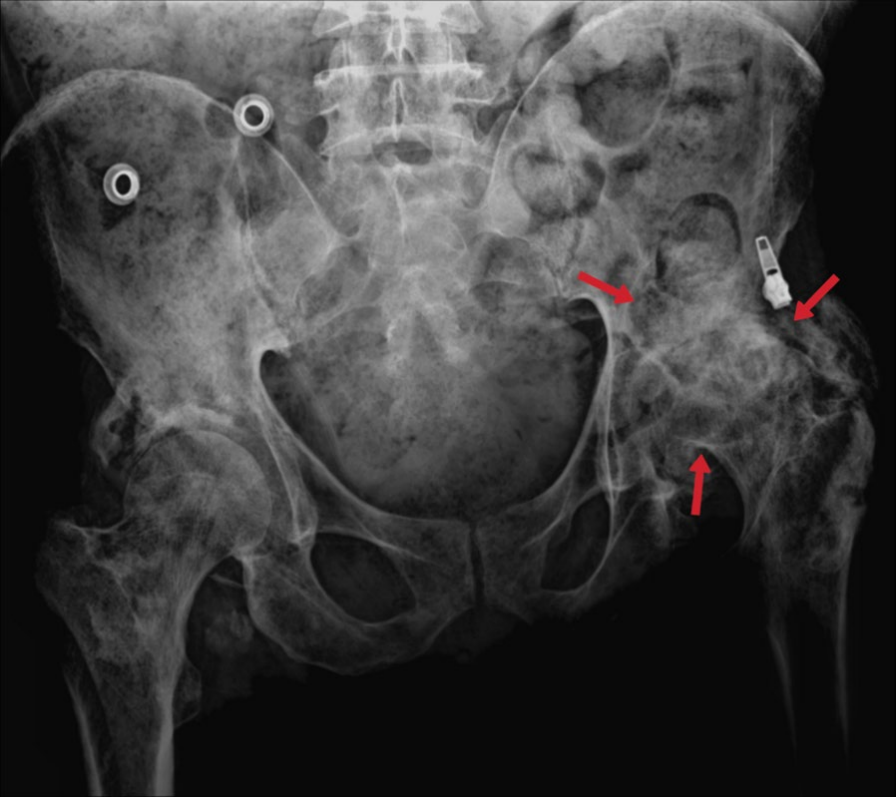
Pelvic X-ray of a 53-year-old female. The left hip joint sequela of TB is characterized by degenerative arthritis (arrows)
